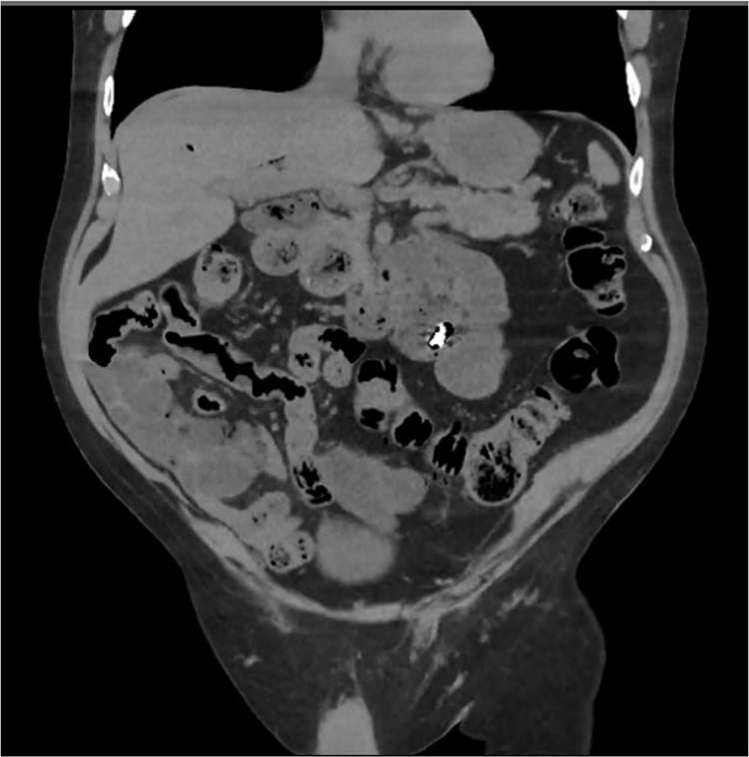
Abdominal CT identifying one hearing aid in a jejunal diverticulum 3 weeks after initial presentation – coronal view.
